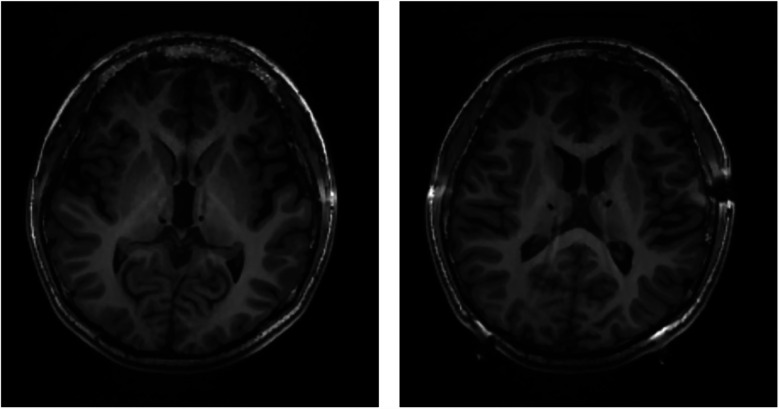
DBS in the CMN of the thalamus in a pediatric patient with drug-resistant epilepsy. Postoperative MRI scans from Patient 1 for visualization of DBS lead placement. DBS leads can be seen as black circles targeting the centromedian nucleus of the thalamus (CMN). The left image is dorsal to the right image.
